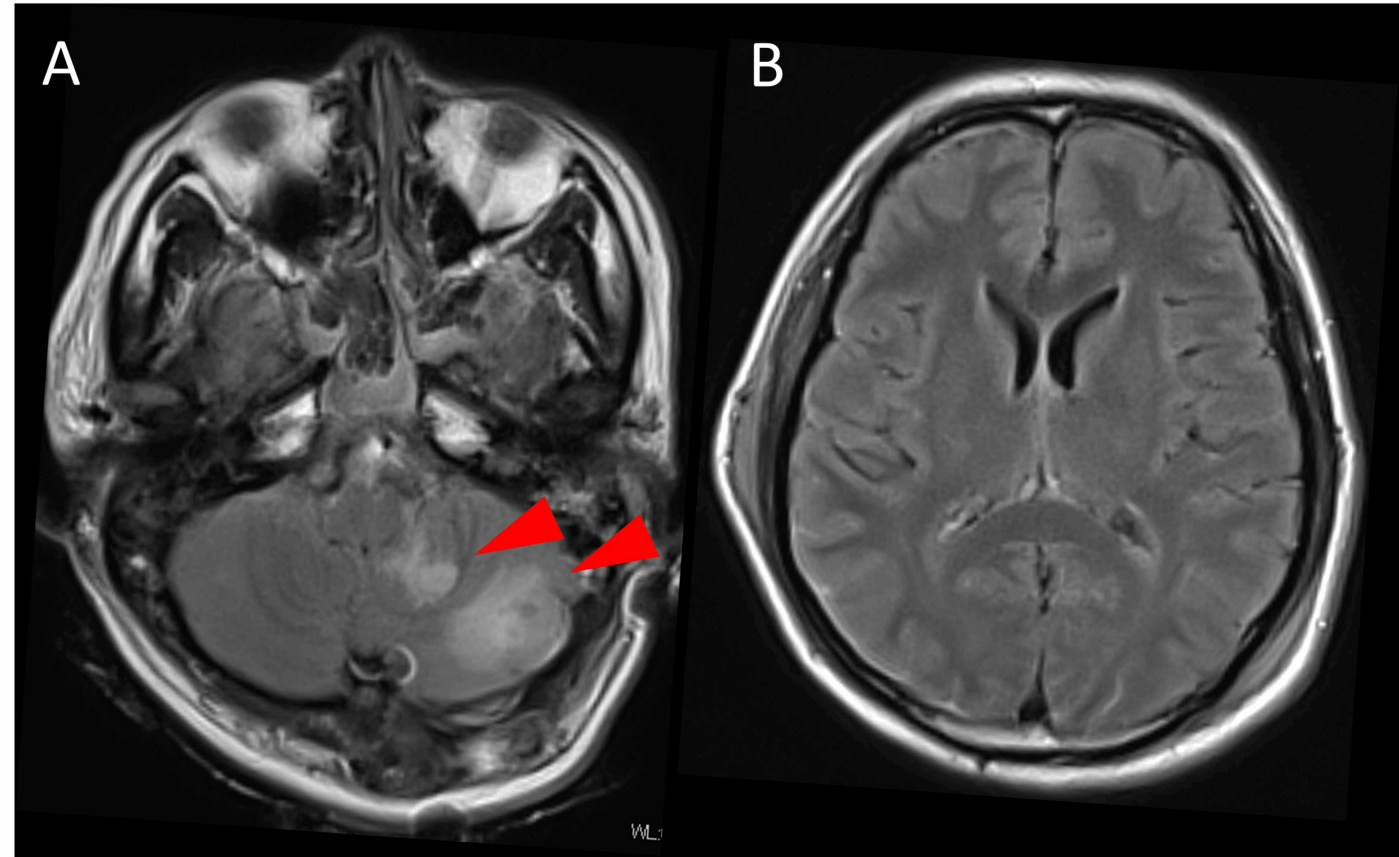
Postoperative ischemic changes and ventricular size. (A) Follow-up CT showing a well-demarcated infarction in the left posterior inferior cerebellar artery (PICA) territory (arrowheads). (B) No ventricular enlargement is observed.
